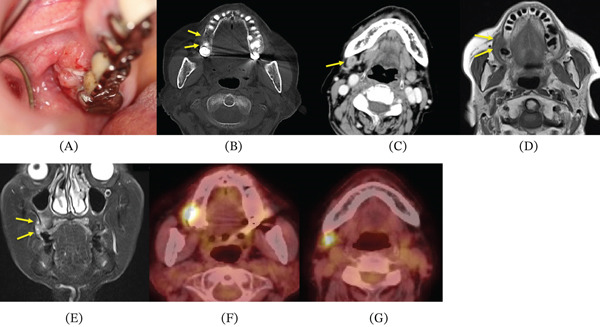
Preoperative imaging. (A) An inward‐growing ulcer measuring 25 × 10 mm is observed, mainly on the buccal gingiva in the right maxillary molar region (Teeth 8‐7‐6). (B) Contrast‐enhanced computed tomography (CT) shows irregular resorption of the buccal alveolar bone in the right maxillary molar region (8‐7‐6). (C) Enlarged right submandibular lymph node (10 mm). Magnetic resonance images show low and high signal intensities on (D) T1‐ and (E) T2‐weighted images, respectively, with evidence of invasion of the buccinator muscle. Positron emission tomography‐CT images show increased fluorodeoxyglucose uptake in the (F) right maxillary molar region and (G) right submandibular lymph node.
